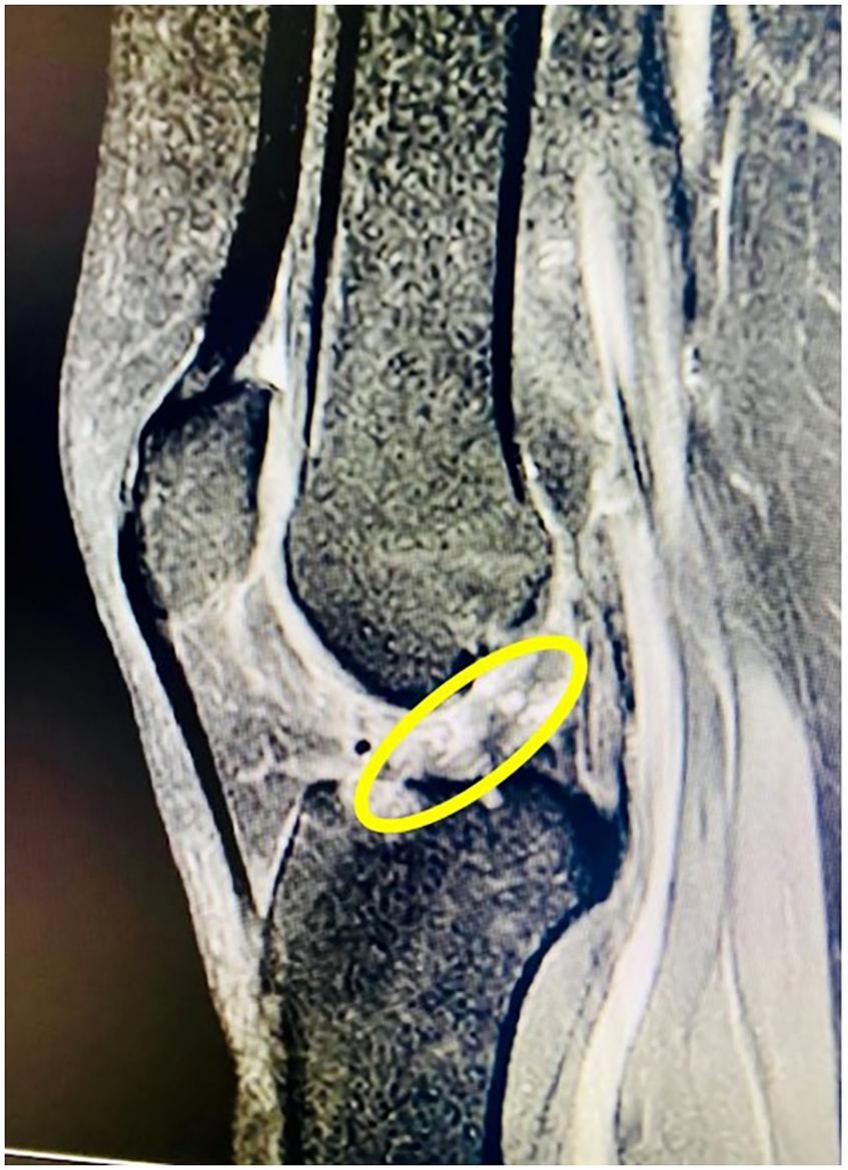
Pre-operative MRI showing complete ACL rupture in a paraplegic patient.
